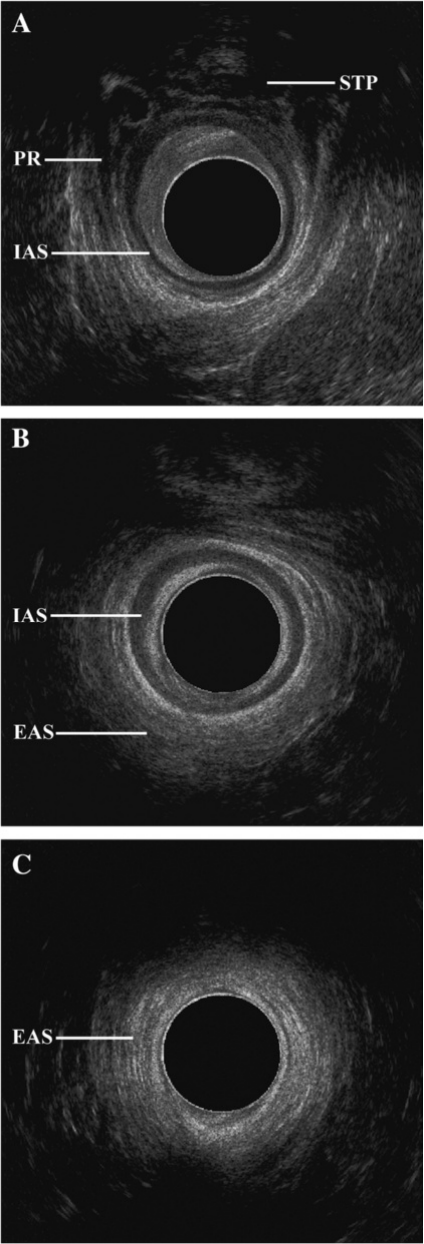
Endosonographic morphology of the anal canal: a high level (A) at the level of the sling of the puborectalis muscles (PR) and superficial transverse perineal muscles (STP), mid level (B) with a well-formed internal (IAS) and external anal sphincter (EAS), and superficial/low level (C) with the external anal sphincter.
